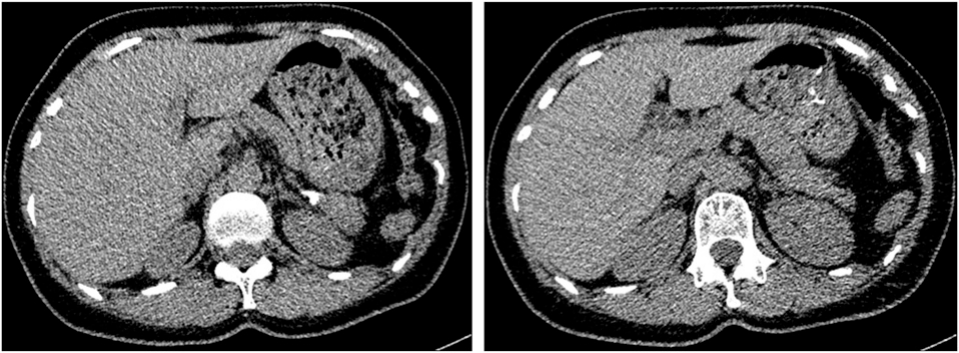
Computed tomography at postoperative 1-year follow-up showing the ligation nail image of splenic hilus underwent by endoscope linear cutting stapler (on left) and no recurrence of hydatid cyst (on right).
